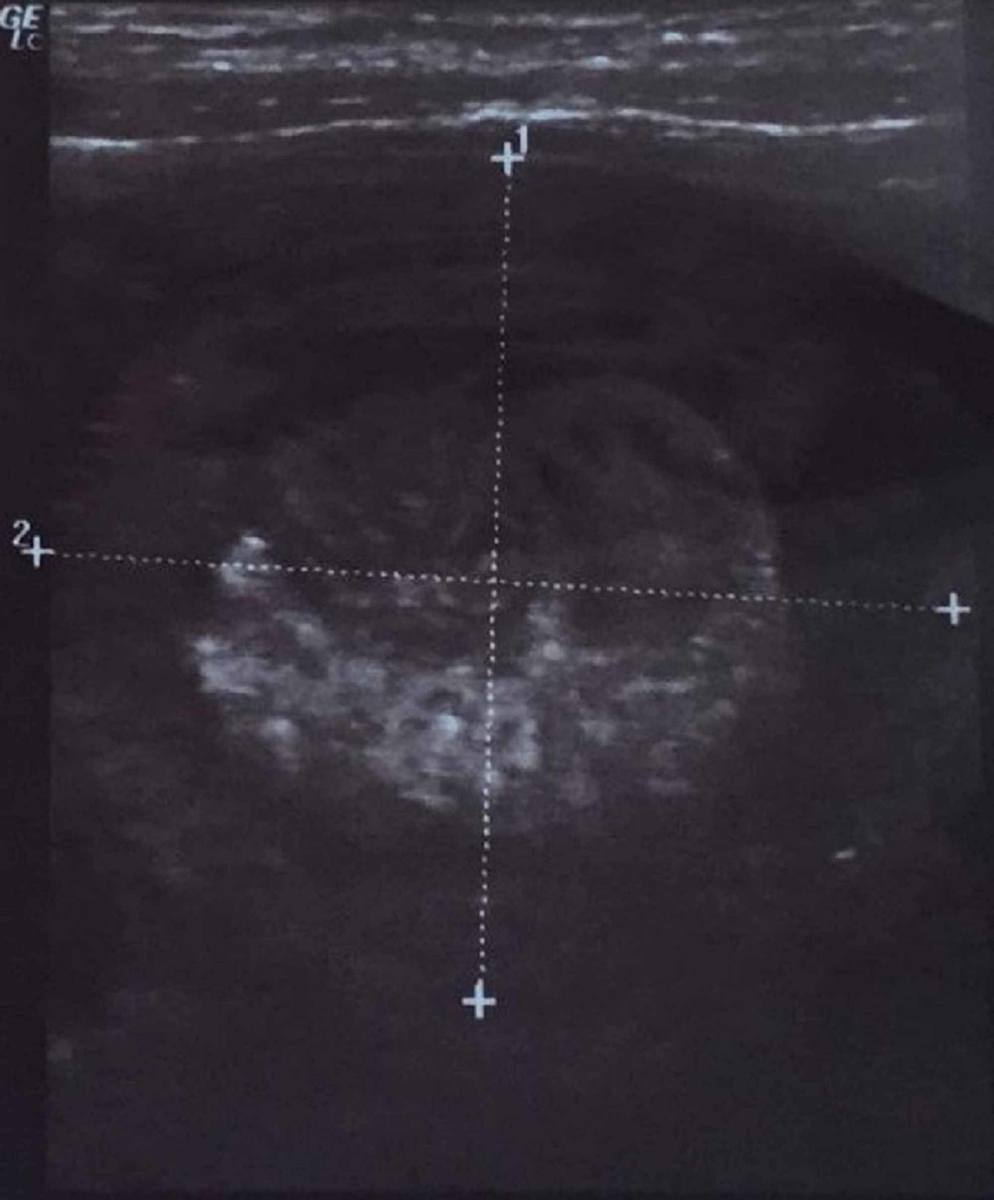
Abdominal transverse ultra-sonographic image showing target type lesion with concentric hypoechoic and hyperechoic layers.
